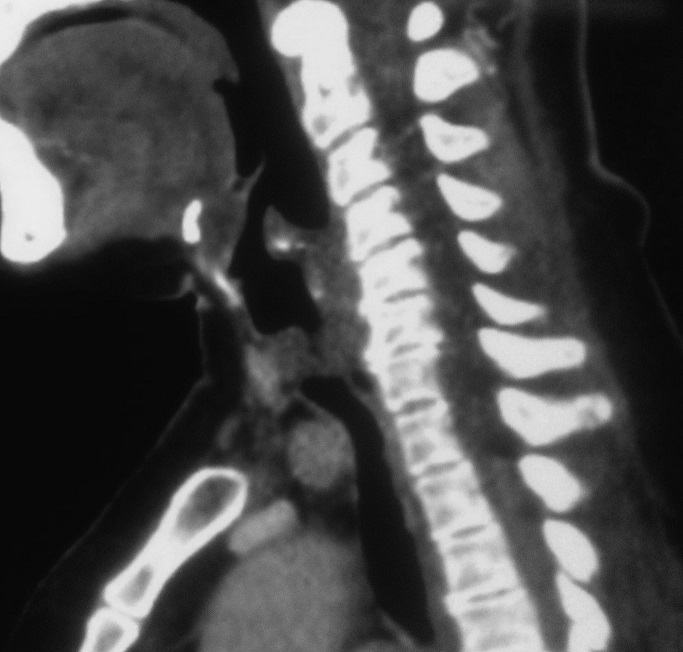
Sagittal reformatted CT images of neck show longitudinal extent of tumor located at upper end of trachea
